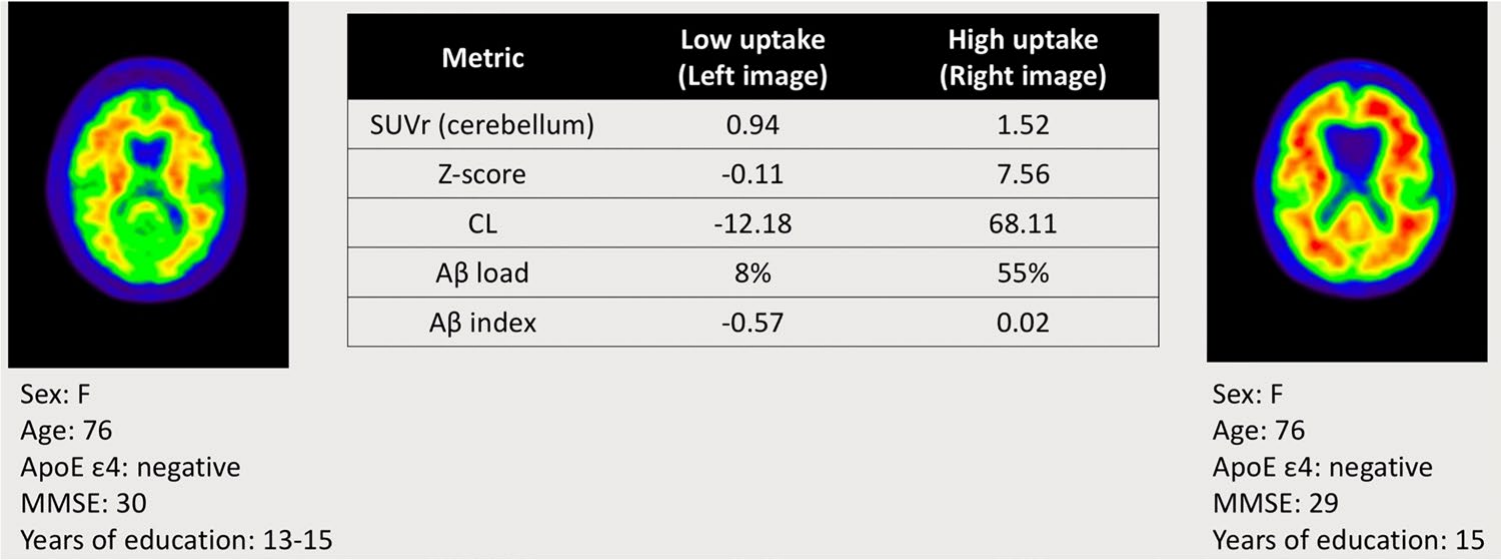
Example of quantitative metrics computed on two subjects from the AIBL dataset scanned with [^18^F]flutemetamol. Low amyloid uptake (left image) and high amyloid uptake (right image), including demographics. It was not possible to compute AMYQ due to the proprietary nature of the software. Abbreviations: mini-mental state examination (MMSE), standardised uptake value ratio (SUVr); amyloid-β (Aβ)
